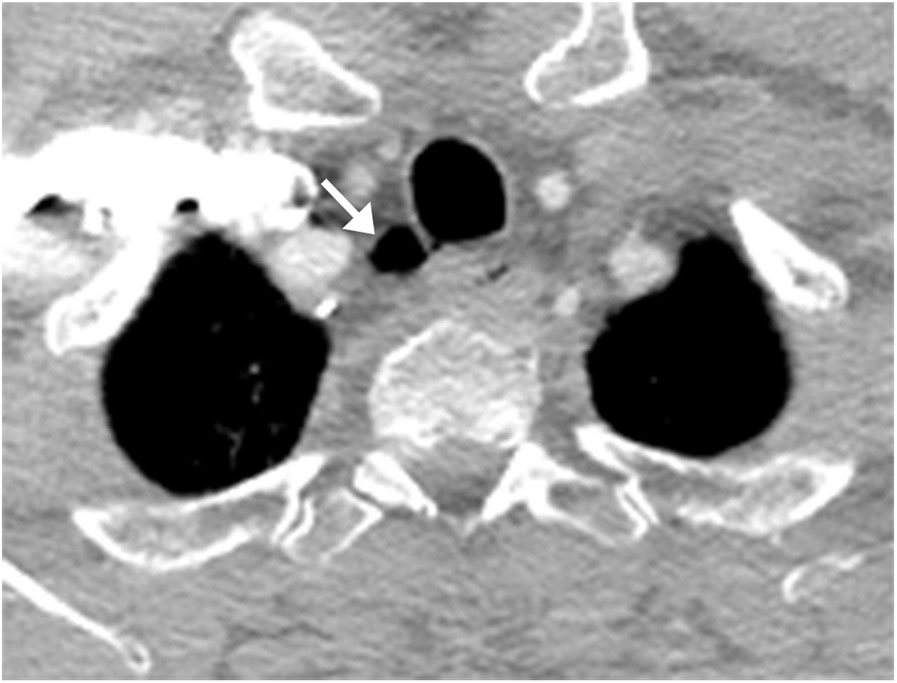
Tracheal diverticulum. Axial CT image demonstrates a focal outpouching (arrow) of the posterior right tracheal wall containing gas, consistent with a tracheal diverticulum
